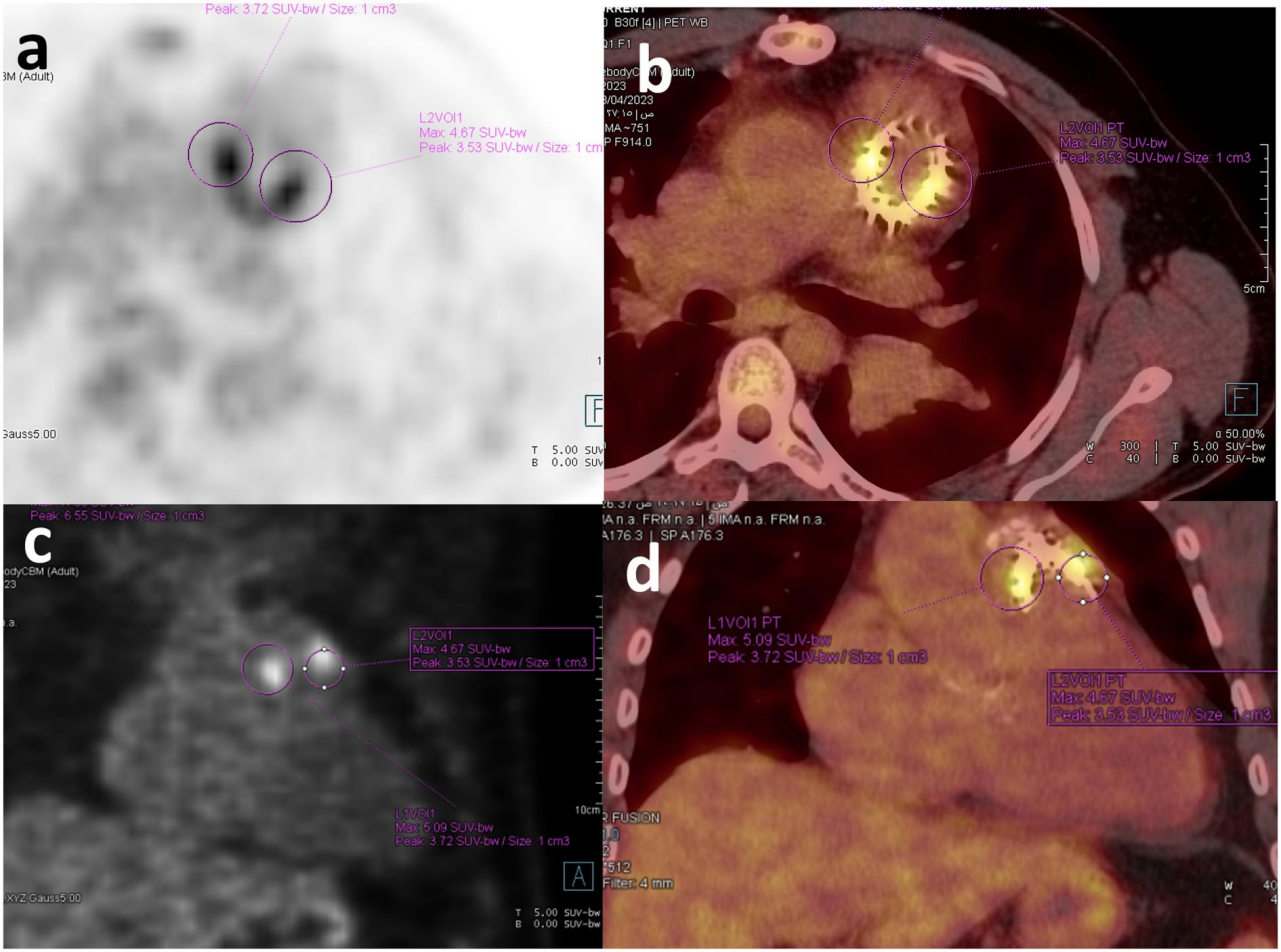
Axial (A) and coronal (C) PET images show two intense foci of increased FDG uptake seen to correspond to the prosthetic valve on the fused images (B and D).
